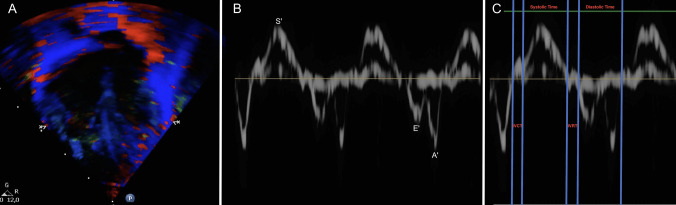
A Tissue doppler imaging (TDI) in the apical four-chamber view. Myocardial velocities are conventionally measured at the septal and lateral insertion points of the mitral and tricuspid valve for septal, right and left ventricle, respectively. B Measurement of peak systolic velocity (s′) and the two diastolic peak velocities—early diastolic (e′) and late diastolic or atrial (a′) waves—corresponding to passive and active ventricular filling, respectively. C Measurement of the time intervals of the four phases of the cardiac cycle: isovolumic contraction time (IVCT), ejection time (ET), isovolumic relaxation time (IVRT), and ventricular filling duration. (FT)
